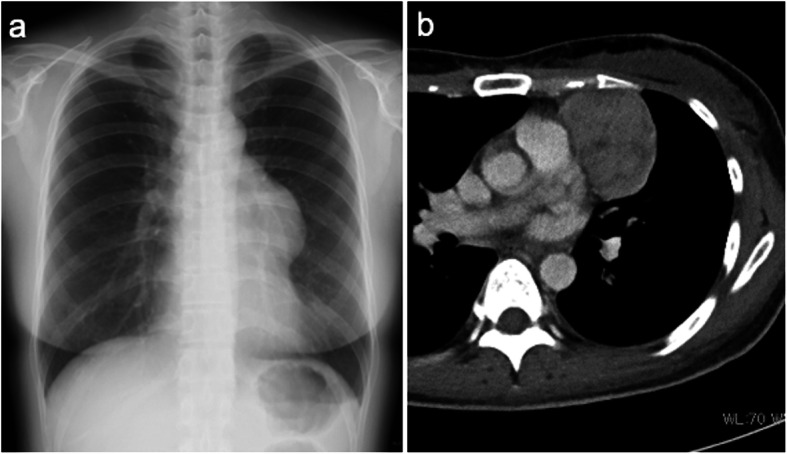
Chest X-ray and Computed tomography on diagnosis. Chest X-ray showing a mediastinal tumor protruding into the left chest cavity (a). Chest computed tomography scan showing a well-defined 61 × 45-mm tumor (b)
